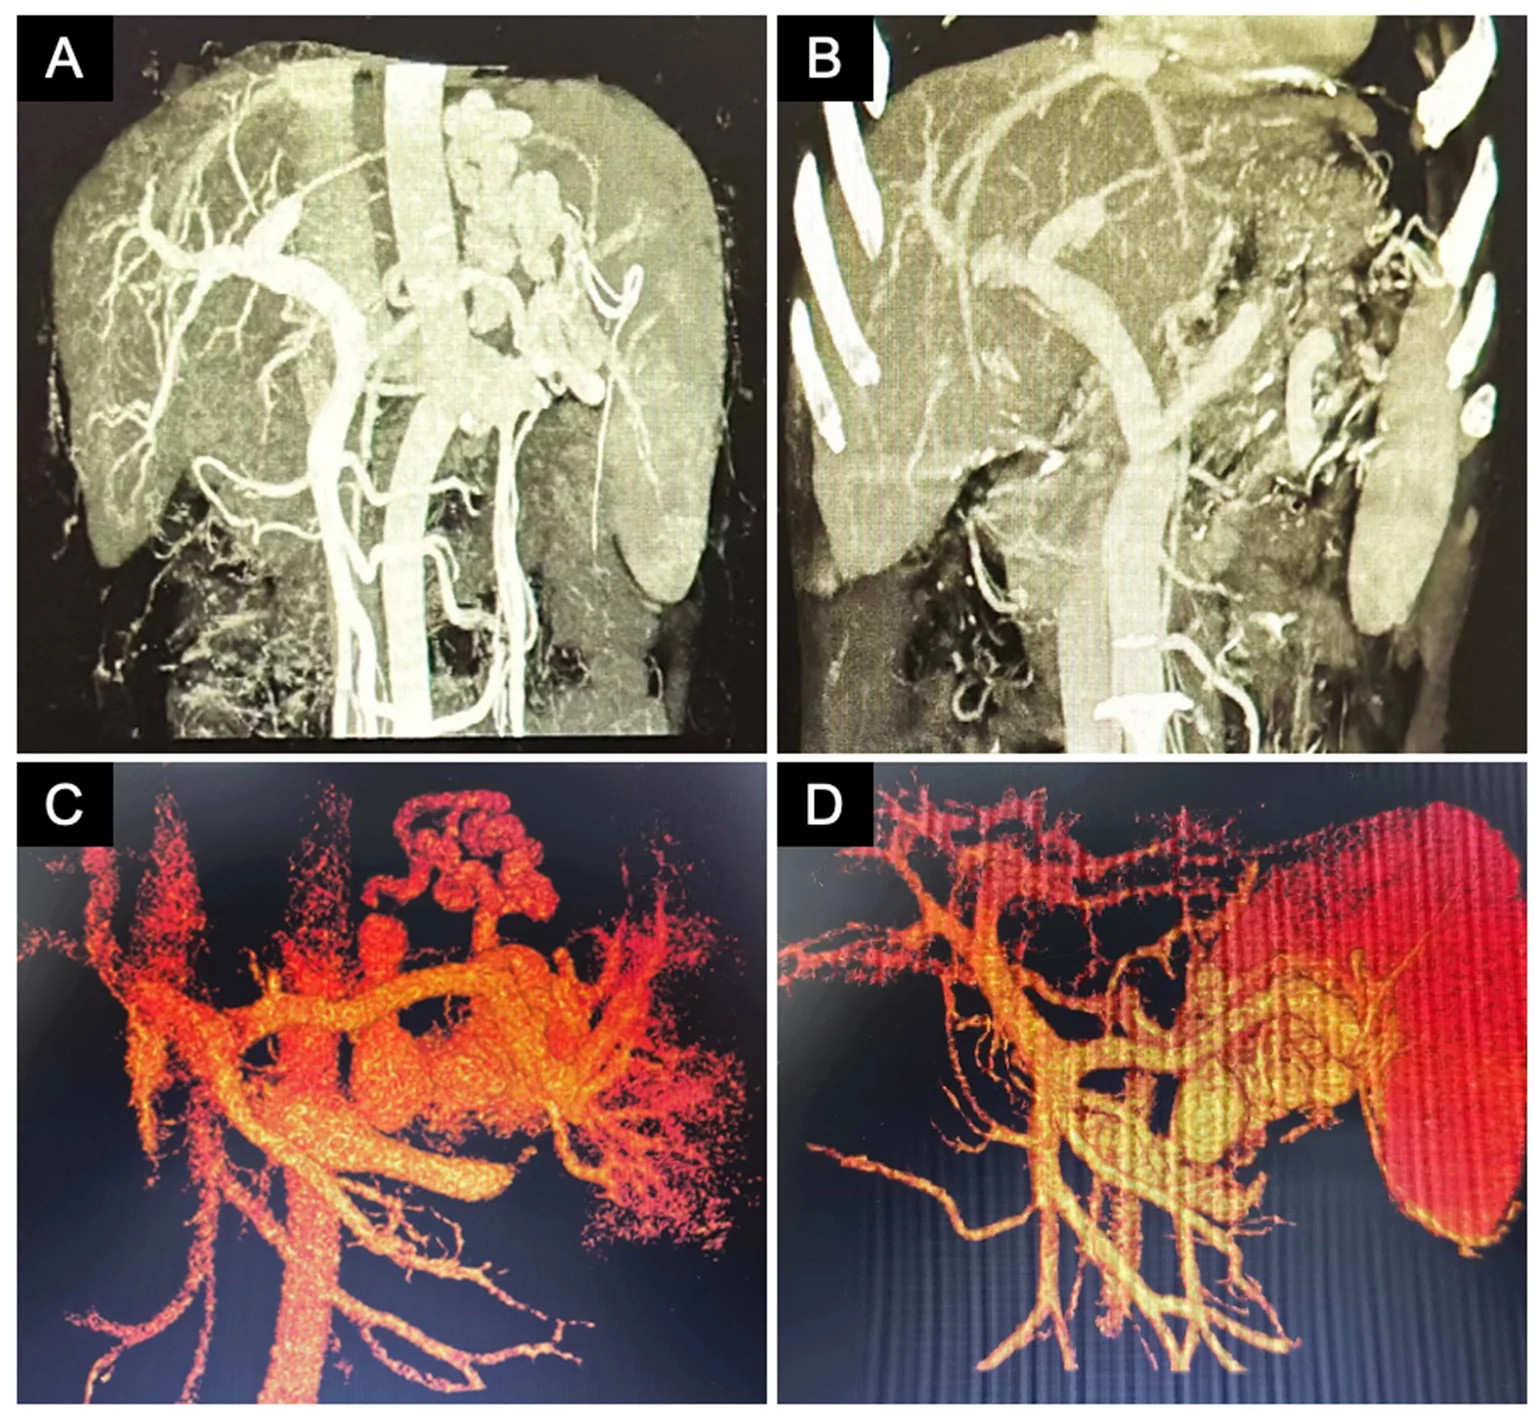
Representative imaging changes in large spontaneous portosystemic shunts before and after endoscopic cyanoacrylate injection. (A) and (B) Coronal CT angiographic images of a large gastrorenal shunt before (A) and after (B) endoscopic cyanoacrylate injection. (C) and (D) Three-dimensional vascular reconstruction images of a large splenorenal shunt before (C) and after (D) glue injection.
